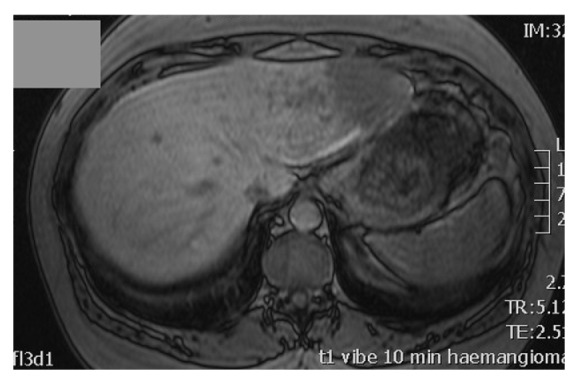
MRI scan showing abnormal areas in segments two, three, and four of the liver.
